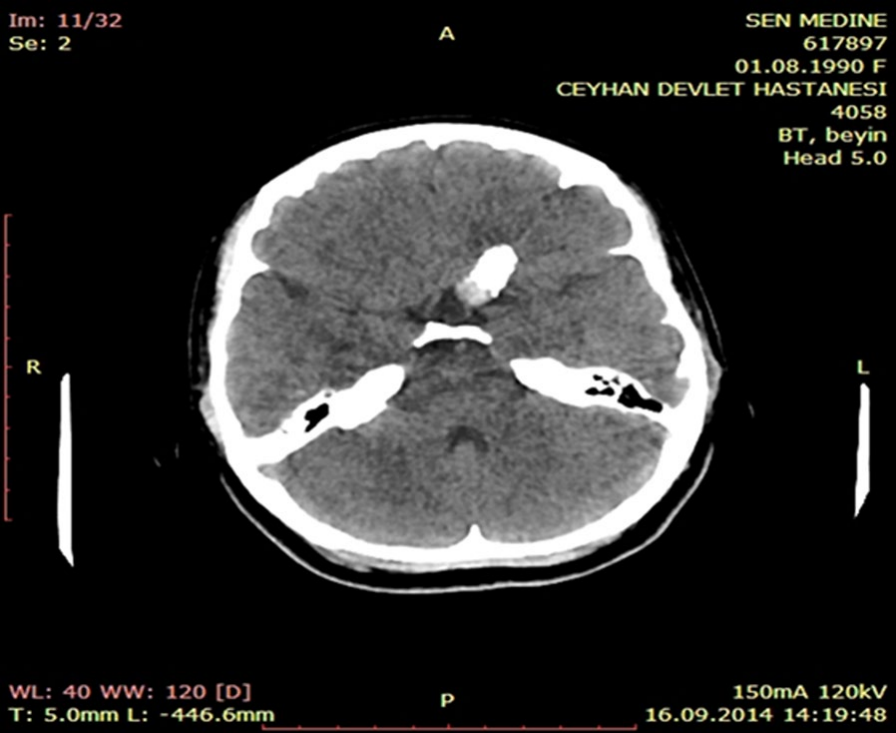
Cranial CT view of the formation
